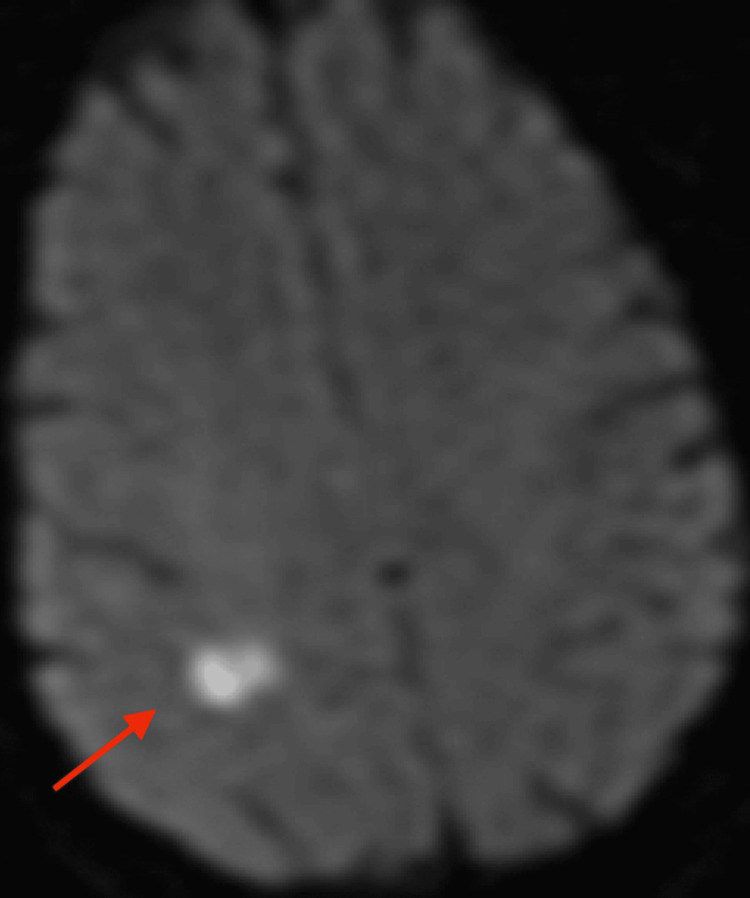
Brain MRI DWI axial Acute infarct involving high right parietal subcortical white matter. DWI: Diffusion-weighted imaging
